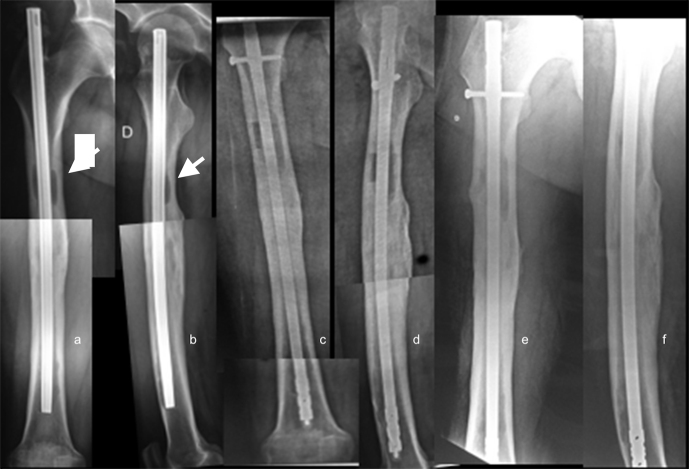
(a, b) AP and lateral radiographs of a patient with a
